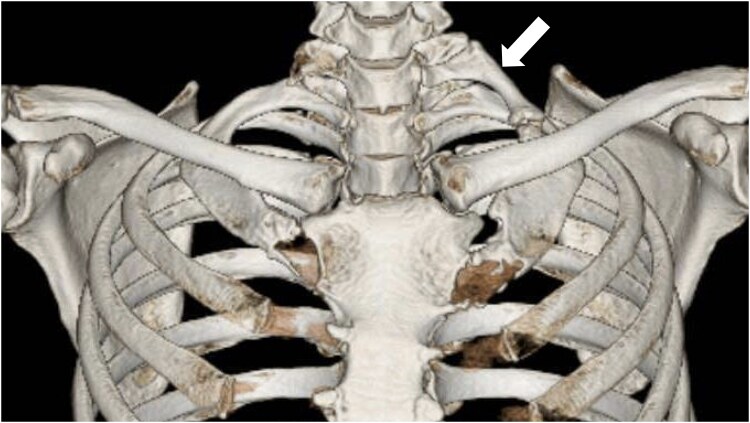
Computed tomography revealed pseudarthrosis due to left cervical ribs (white arrow).
